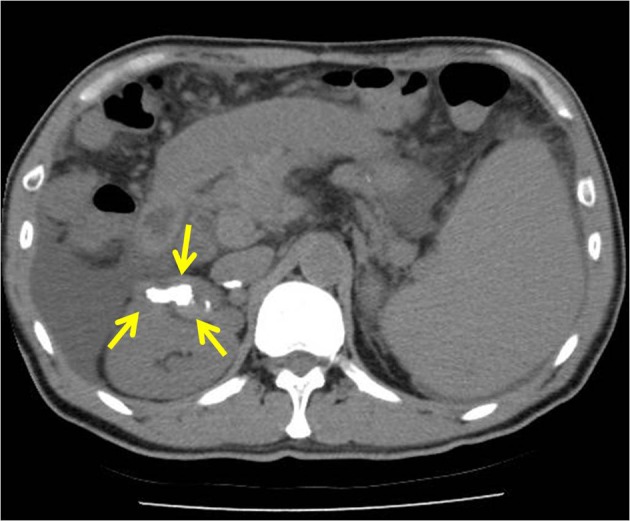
Computed tomography scan shows properly obliterated collateral vessels with N-butyl-2-cyanoacrylate and lipiodol (arrows).
